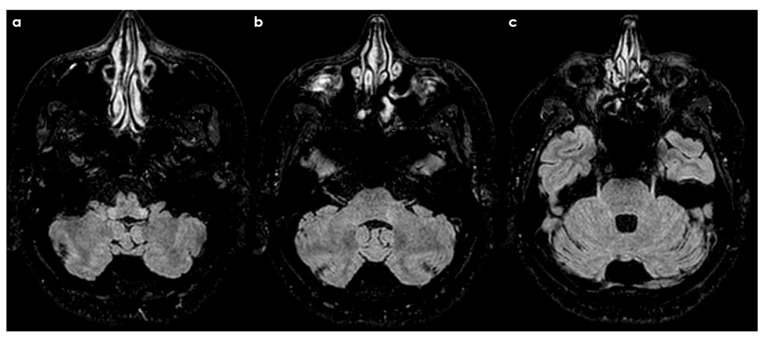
Three-month follow-up MRI of the same patients as in Figure 1 and Figure 2, after steroid treatment, showing a strong reduction in pontine hyperintensities with persisting hyperintense trigeminal tract in ascending axial FLAIR slices from (a–c).
